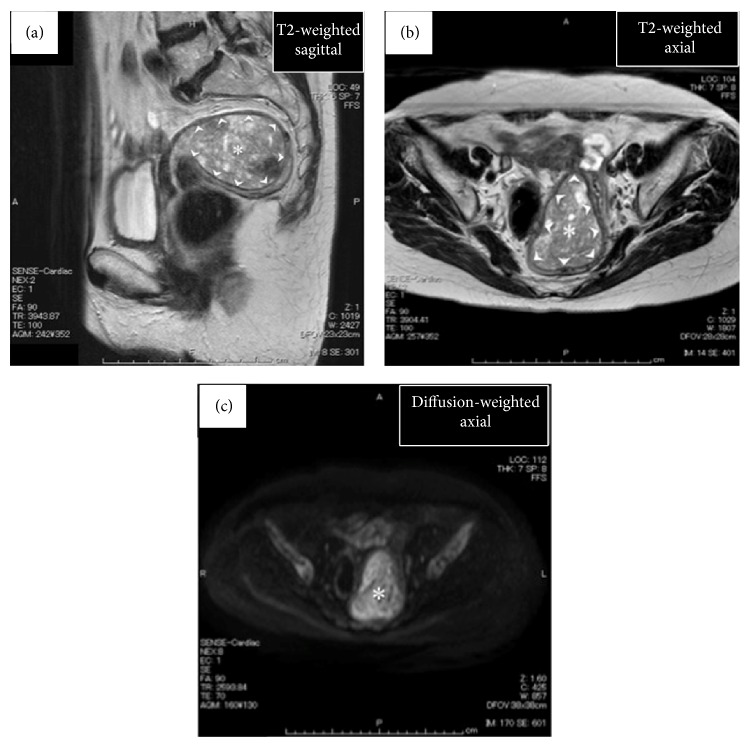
Findings of pelvic magnetic resonance images (MRI). ((a) and (b)) Sagittal (a) and axial (b) planes in T2-weighted MRI. Uterine cavity is filled with heterogeneous polypoid tumor (∗). Note the smooth boundary between the tumor and the thinned myometrium (arrowheads). (c) Axial plane in diffusion-weighted MRI. Uterine tumor (∗) exhibits high signal intensity as compared with the surrounding myometrium.
